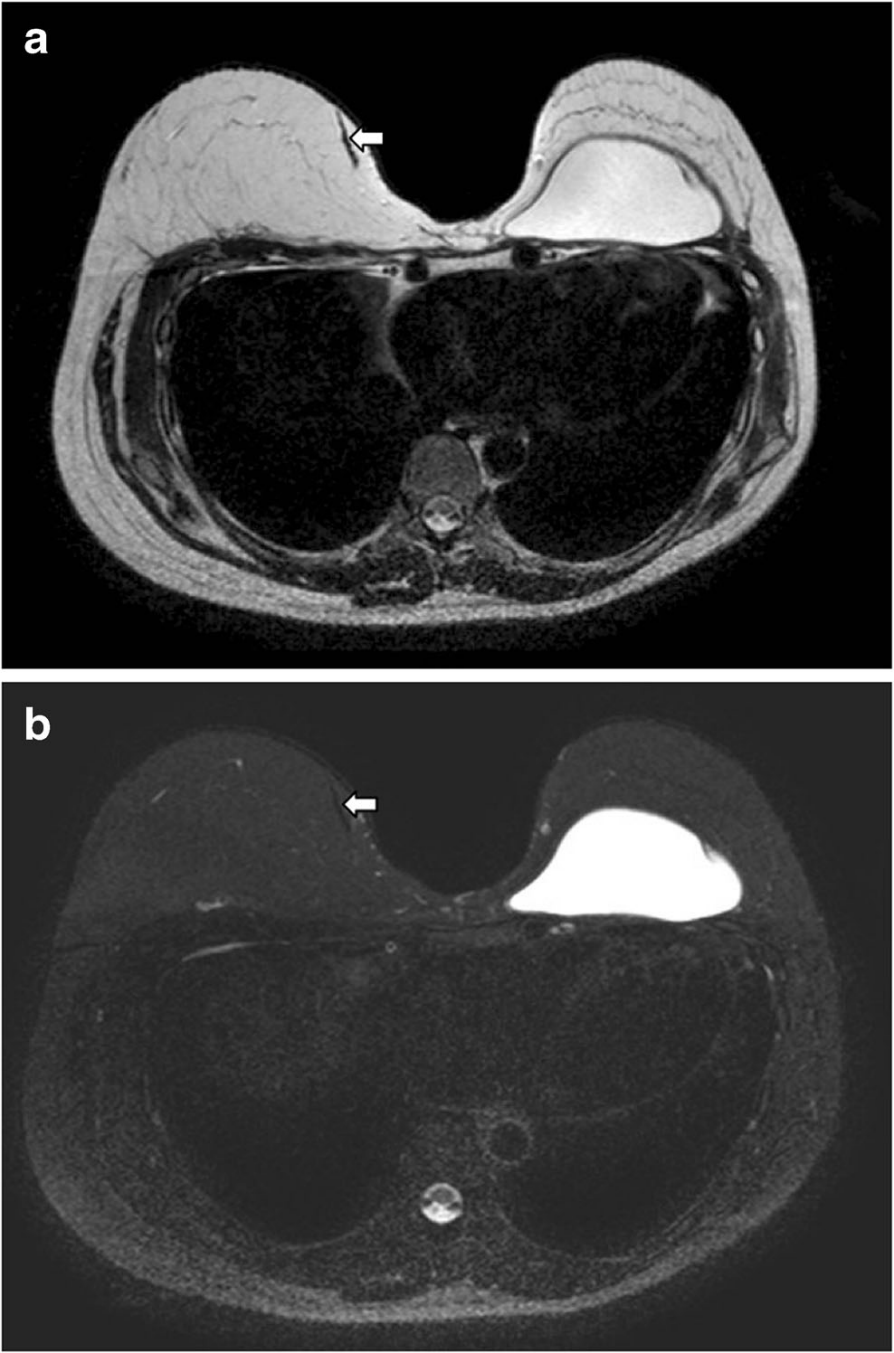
Myocutaneous flaps. These are MR images of a patient with history of bilateral mastectomy and breast reconstruction with TRAM flap at right breast and latissimus dorsi flap plus saline-filled implant at left breast, in order to maintain breasts symmetry. T2-weighted (a) and T2-weighted fat-saturated (b) axial MR images reveal the complete fatty composition of both breasts without normal glandular tissue, except over the region of saline-filled implant. The denuded dermal layer of the abdominal tissue (arrow) is seen parallel to the skin of right breast
